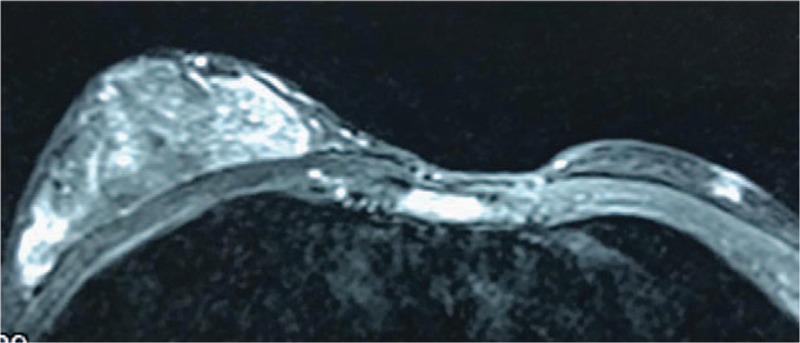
Magnetic resonance imaging (MRI) of unilateral gynecomastia (vertical plane).
